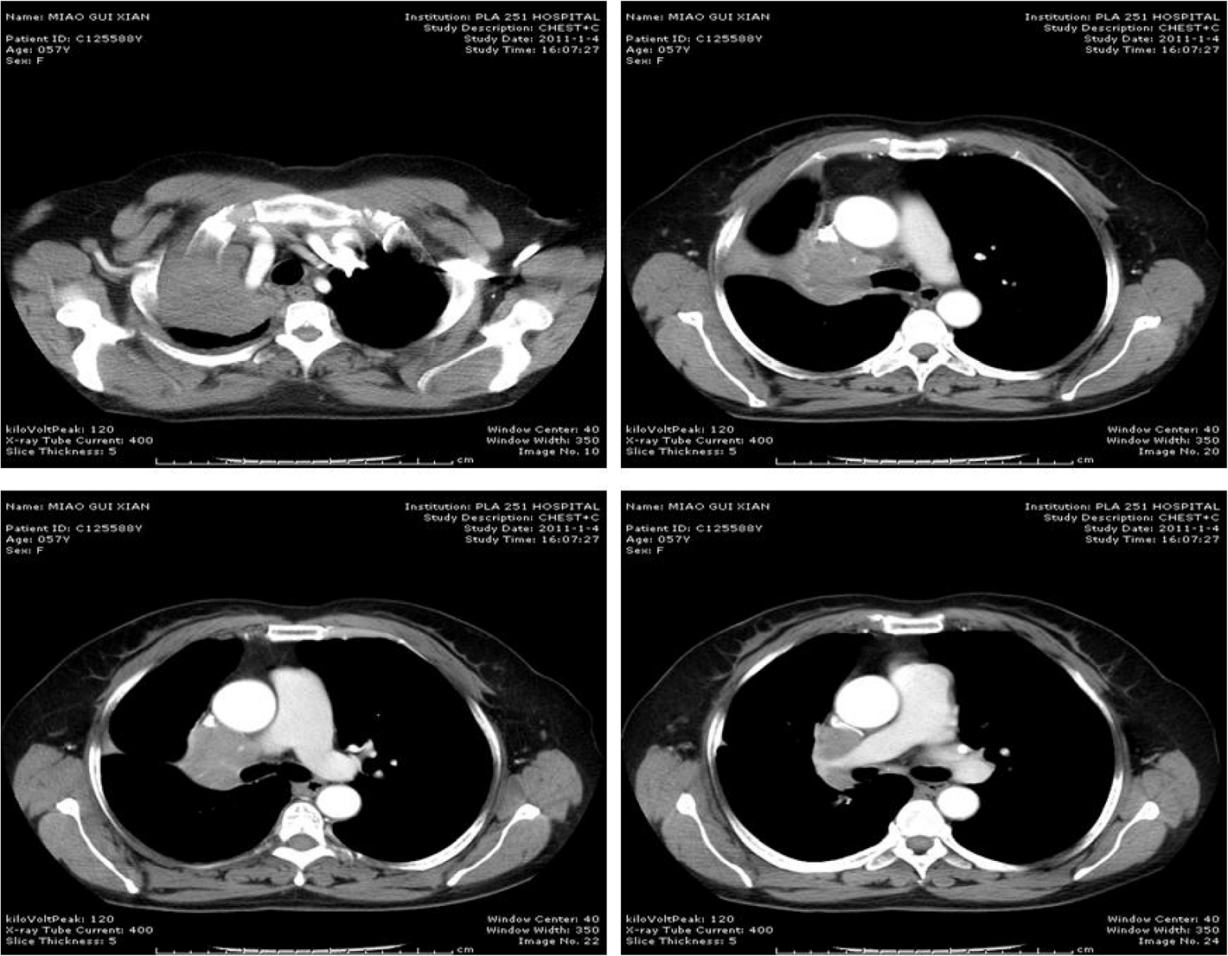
Computed tomography (CT) scan of the chest show atelectasis of right upper lobe tumor invasion into the right main pulmonary artery
